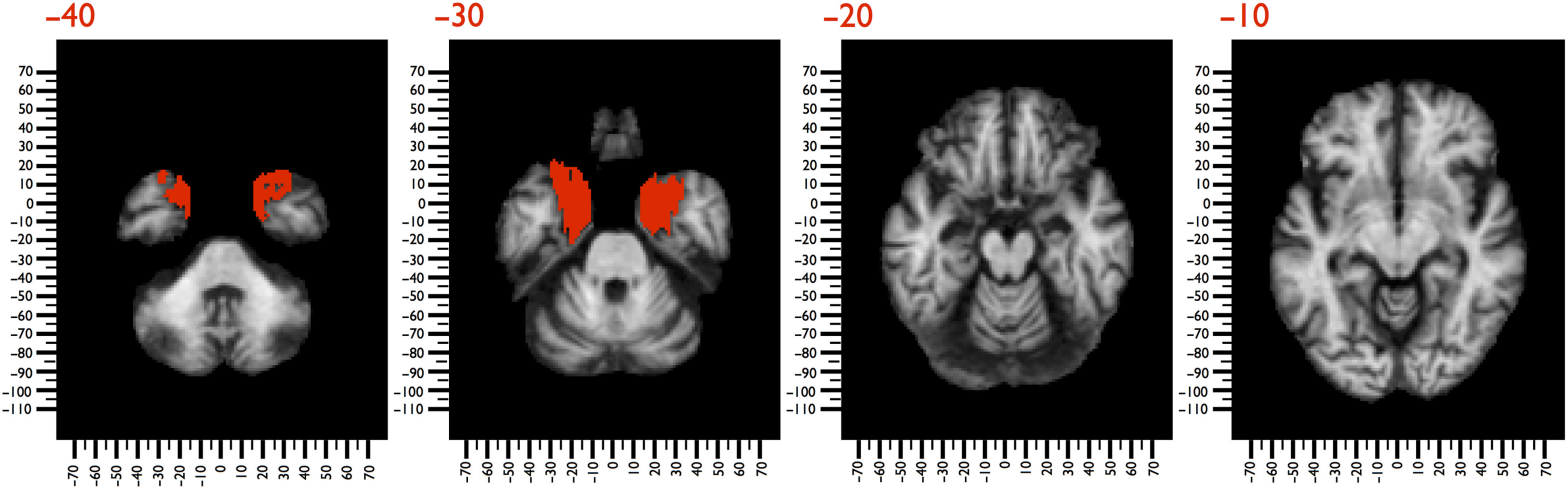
T*_1_-weighted MRI images of Molaison's brain, registered to MNI152 space. The bilateral temporal damage produced by the surgery is shown in red.
